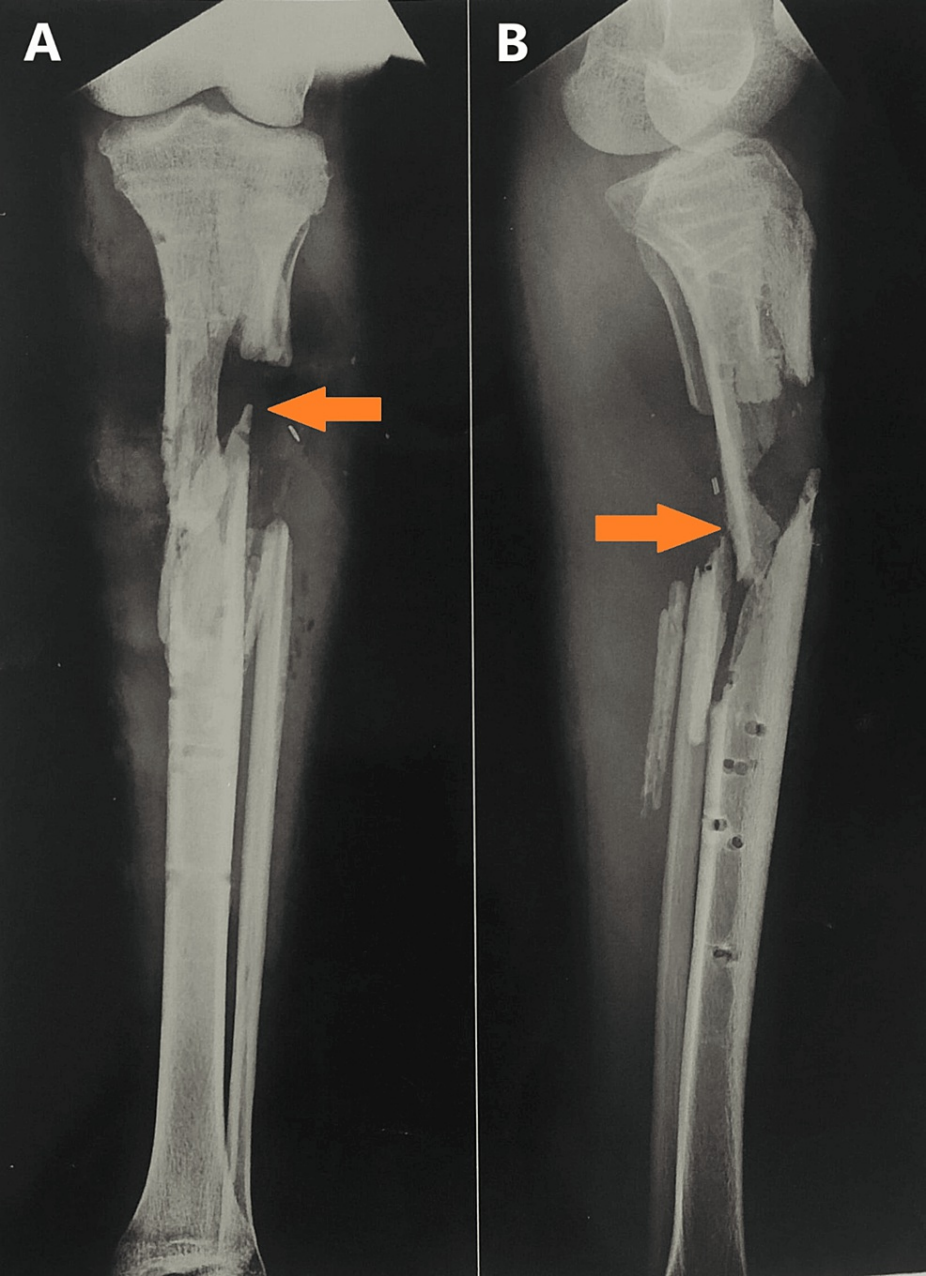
Pre-operative x-ray in anterior and lateral view of compound grade 3c proximal tibia fracture of left lower limb. A. Orange arrow shows a pre-operative x-ray in anterior view of a compound grade 3c proximal tibia fracture of the left lower limb. B. Orange arrow shows a pre-operative x-ray in lateral view of a compound grade 3c proximal tibia fracture of the left lower limb.
